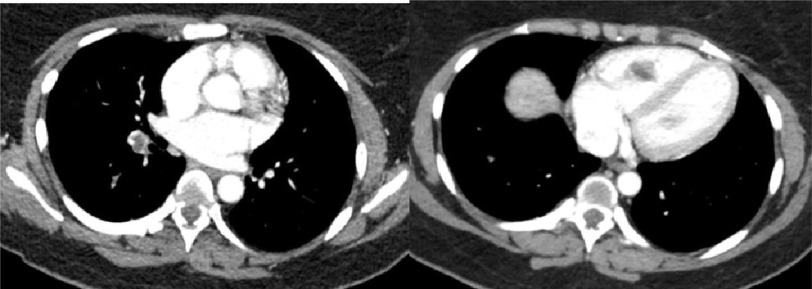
CT angiography shows right greater than left lower lobe pulmonary emboli with right heart strain and slightly increased right lower lobe consolidation from initial CT reflecting pulmonary infarct.
